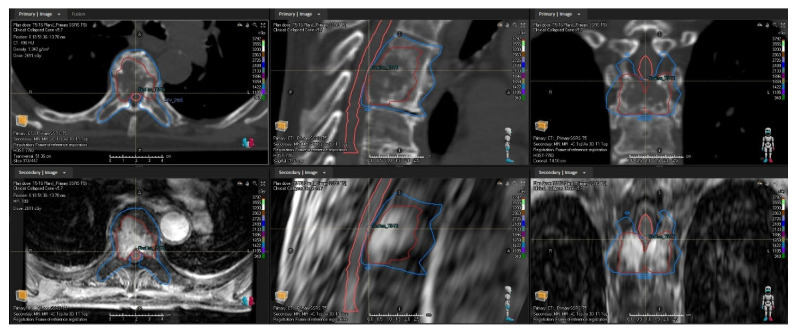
A CT (top row) and T1 + contrast MRI (bottom row) showing the GTV (dark red), CTV (blue), and spinal cord (pink). The treatment targets the 5th and 6th thoracic vertebrae.
